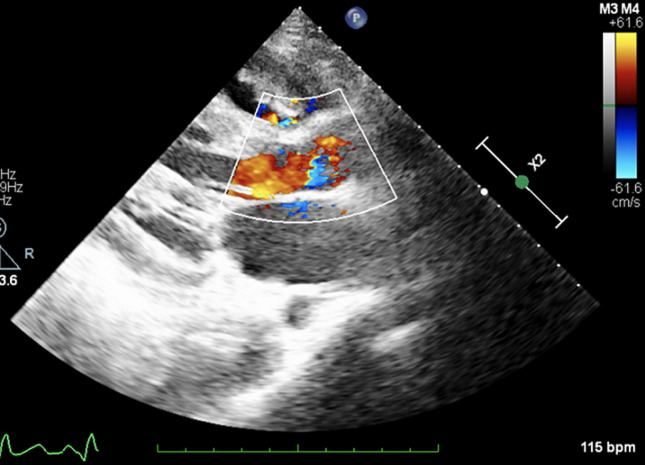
echocardiography showing the vegetation attached to the VSD
